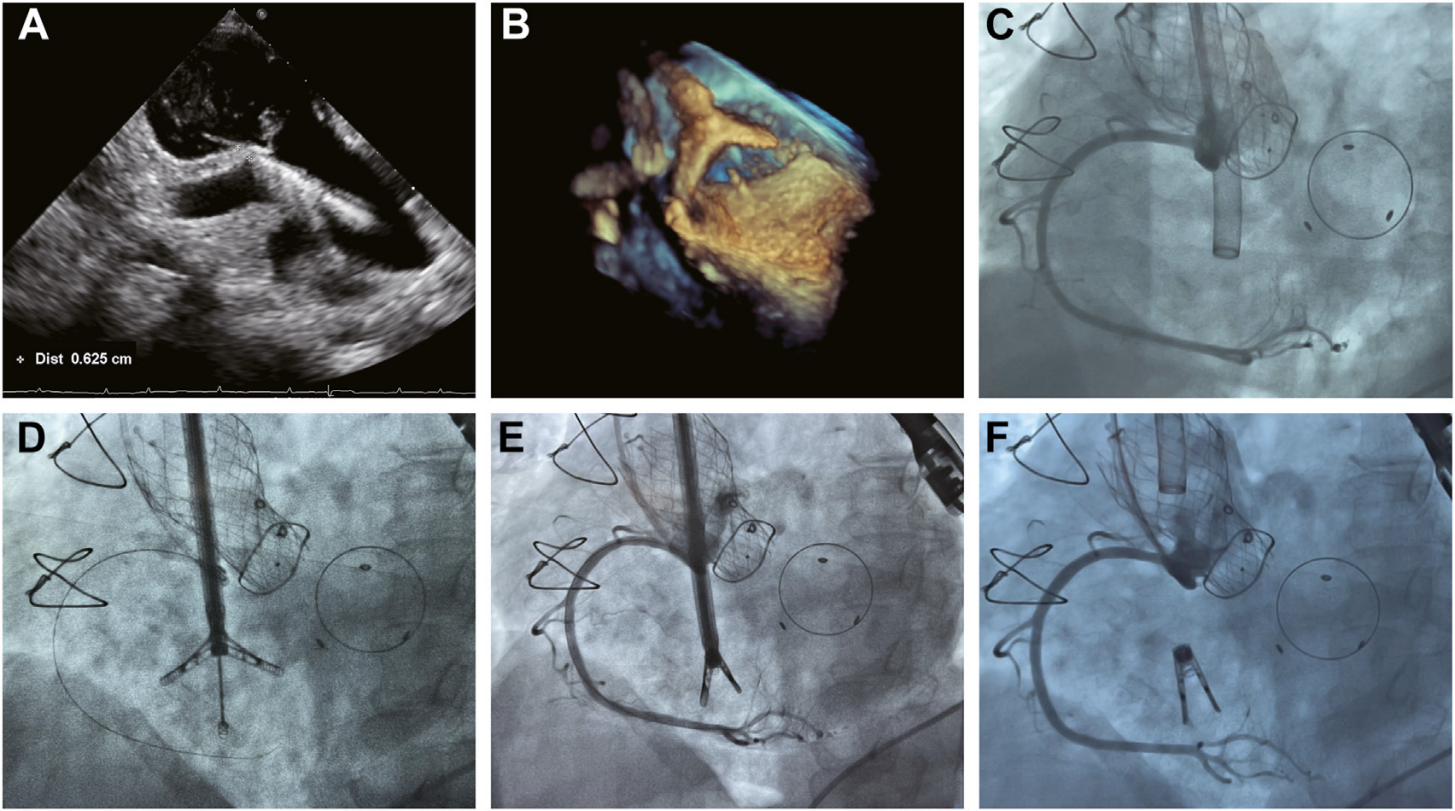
Case Example of K-Clip System Deployment (A) The corkscrew is drilled into the annulus under TTE guidance. (B) The clip arm is rotated to make it flush with the annulus under the 3D guidance of TEE. (C) The deflectable outer sheath is placed, and right coronary angiography is performed. (D) The corkscrew with the annulus tissue is raised outward to the unfolded clip arms. (E) Right coronary angiography is used to evaluate the presence of coronary stenosis and clip stability. (F) After release, angiography is performed again to assess the vascular stenosis. 3D = 3-dimensional; TEE = transesophageal echocardiography; TTE = transthoracic echocardiography.
